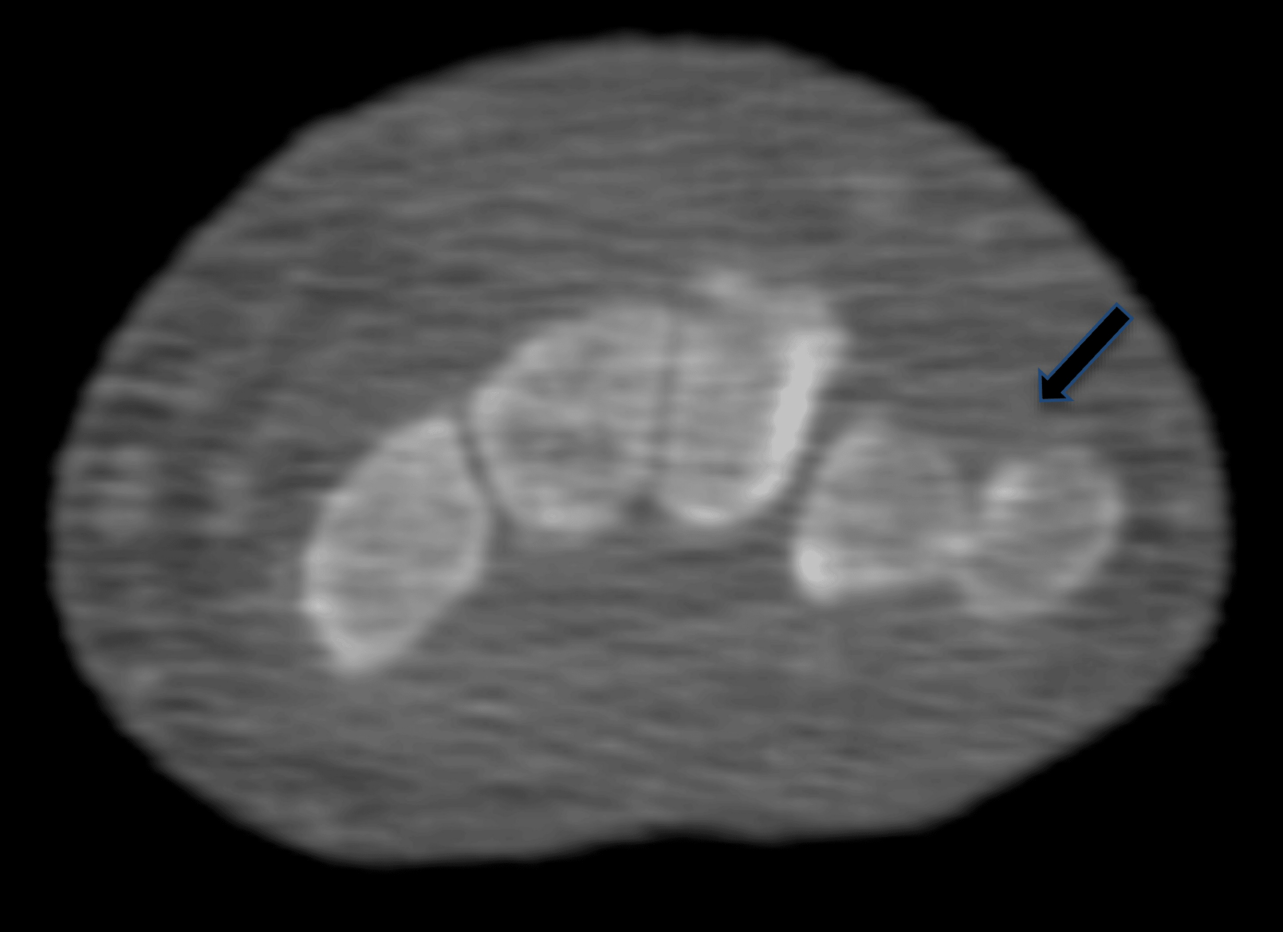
Axial CT demonstrating dorsal ulnar dislocation of the pisiform
